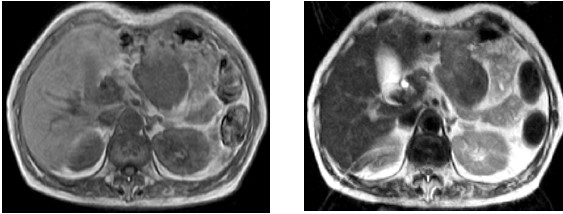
Magnetic resonance imaging (MRI) showed a low signal intensity of the mass in both T1-weighted (left) and T2-weighted images (right).
